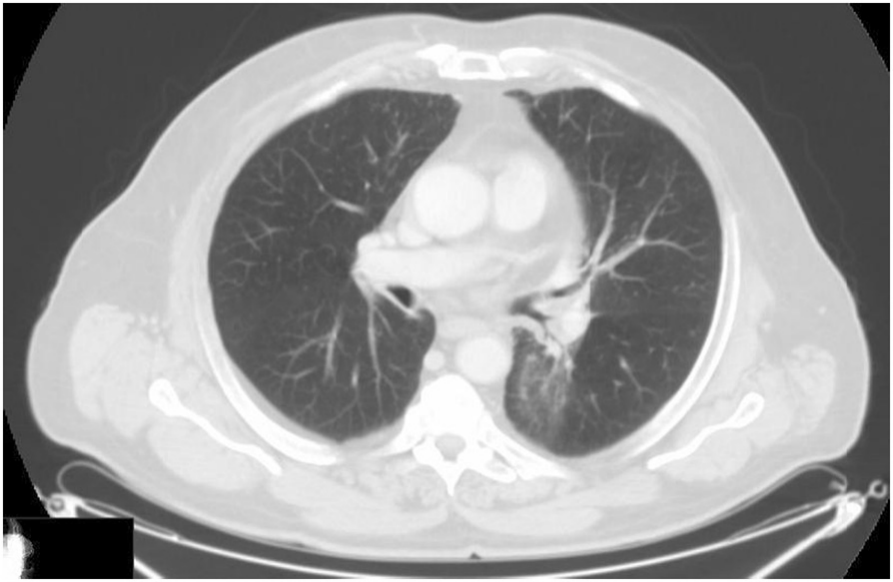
A computed tomography scan performed 1 year after starting treatment with erlotinib and showing a complete response.
